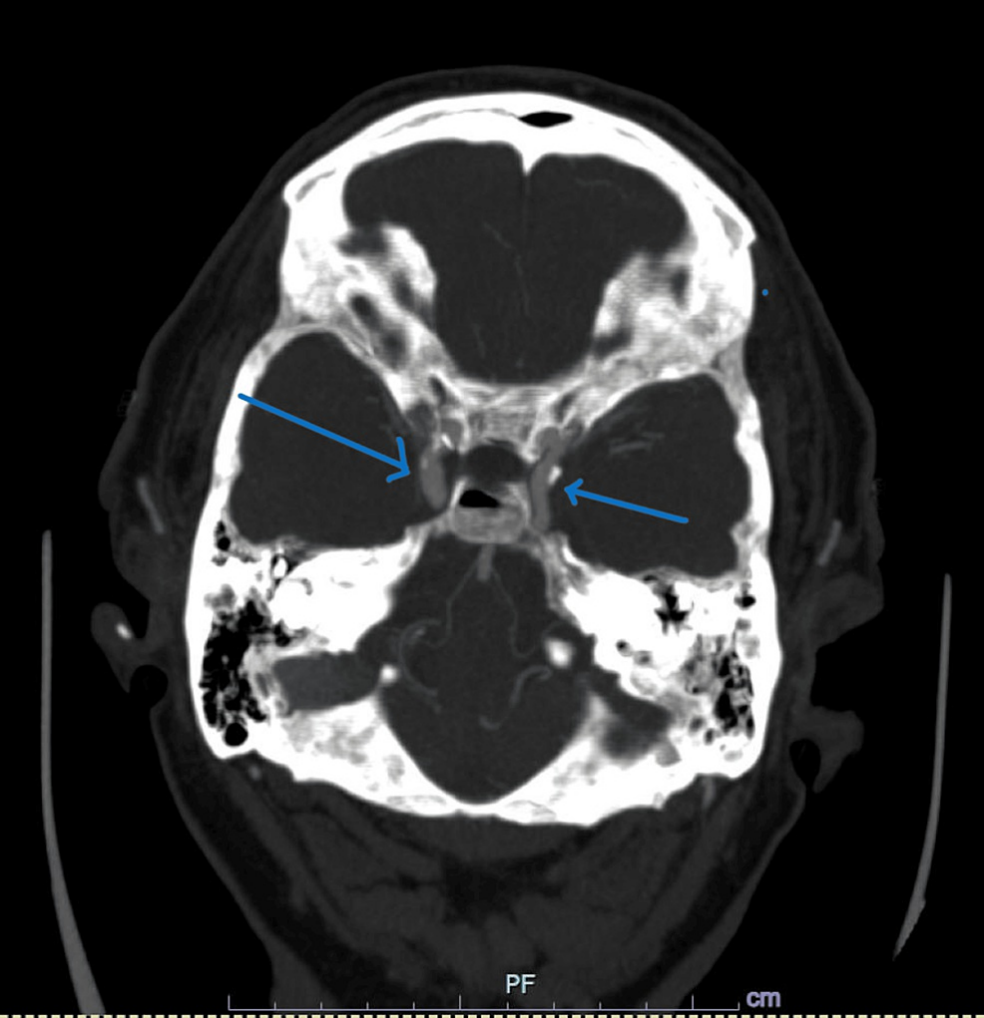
CTA of the head showing enlargement of the ophthalmic veins CTA, computerized tomographic angiography
